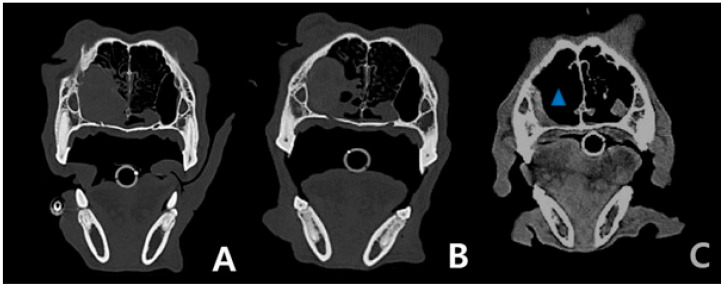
(A–C) Axial view of the CT scan of the skull. The size of the tumor decreased significantly (blue triangle) after radiation/chemotherapy compared with before radiation/chemotherapy, after radiation, and before chemotherapy.
